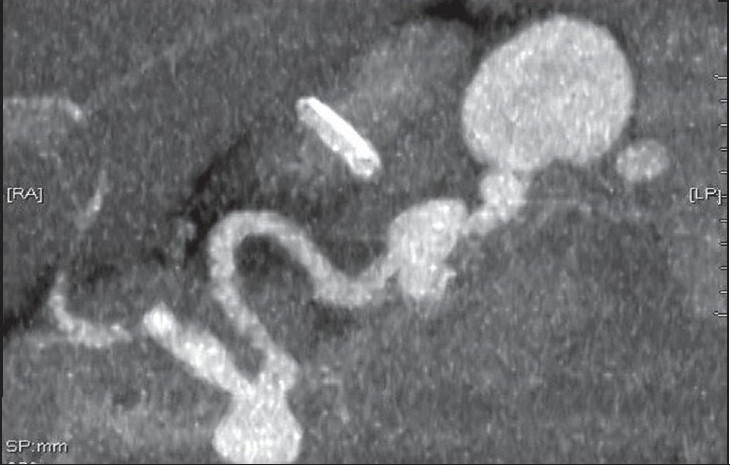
Spiral CT images with intravenous contrast enhancement
